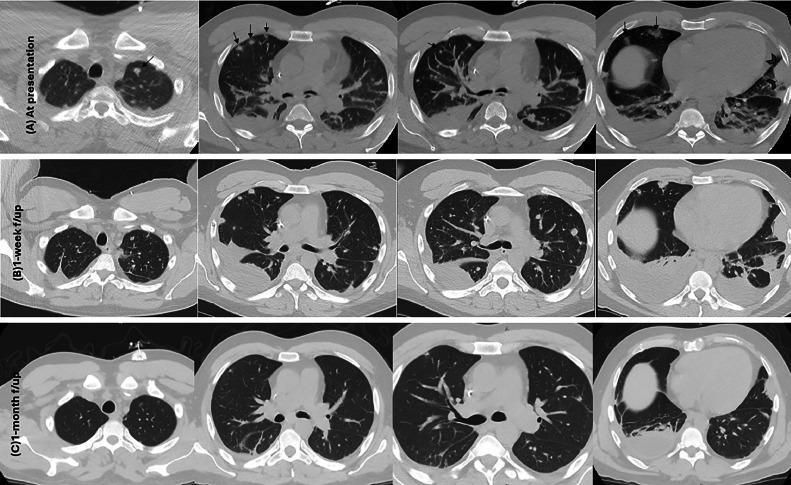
Axial CT images of the chest demonstrate temporal evolution of pulmonary findings. (A) At presentation of respiratory symptoms, multiple poorly defined (ill-marginated) bilateral pulmonary nodules, some with surrounding ground-glass opacity (arrows), lingula consolidative mass (arrow heads) with small bilateral pleural effusions. (B) One-week follow-up CT demonstrates persistent/ slightly increased bilateral pleural effusions with persistent pulmonary opacities. (C) One-month follow-up CT shows marked improvement of the pulmonary nodules, with residual pleural effusions.
